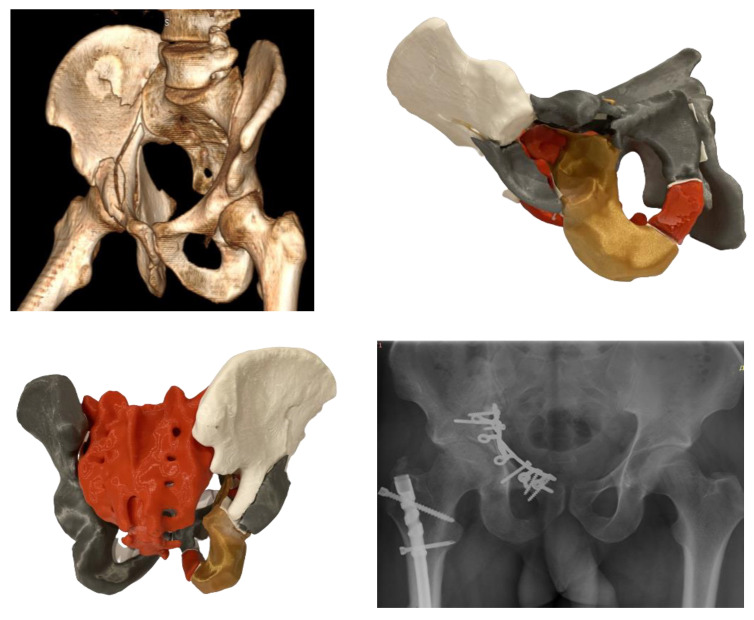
A reconstructed CT scan from a clinical case with substantial comminution of the quadrilateral surface (top left). A corresponding 3D printed pelvis model in (1) inside view, with extracted femoral head for better visualization of the intra-articular acetabular surface (top right), and (2) posterior view (bottom left), demonstrating comminution of both the posterior column and posterior wall. A postoperative radiographic image of the fixation with an omega plate (bottom right).
